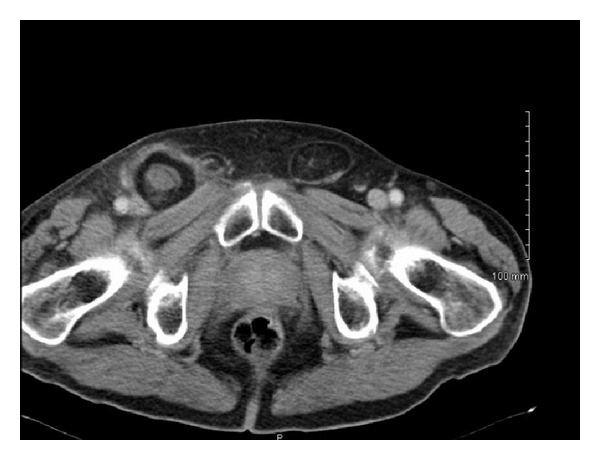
Preoperative CT scan demonstrating incarcerated femoral hernia containing a perforated appendix.
